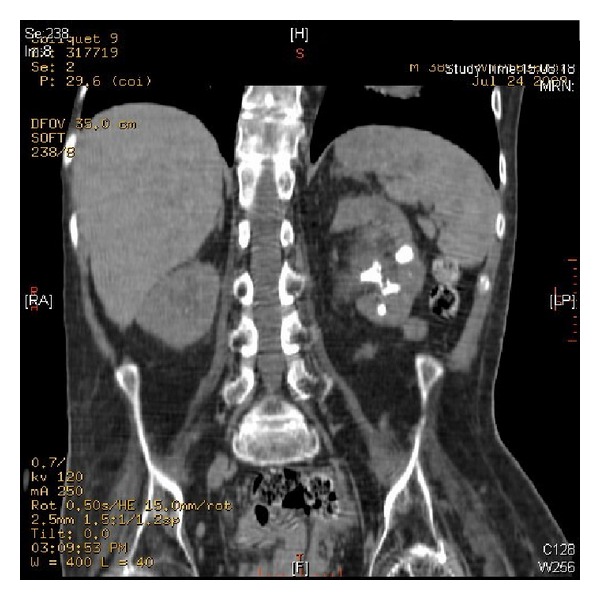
CT of urinary tract, performed on 24 July 2009, revealed multiple calculi in left kidney.
